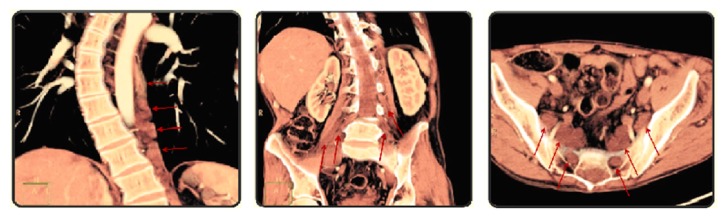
Abdominal CT scan showing neurofibromas.
